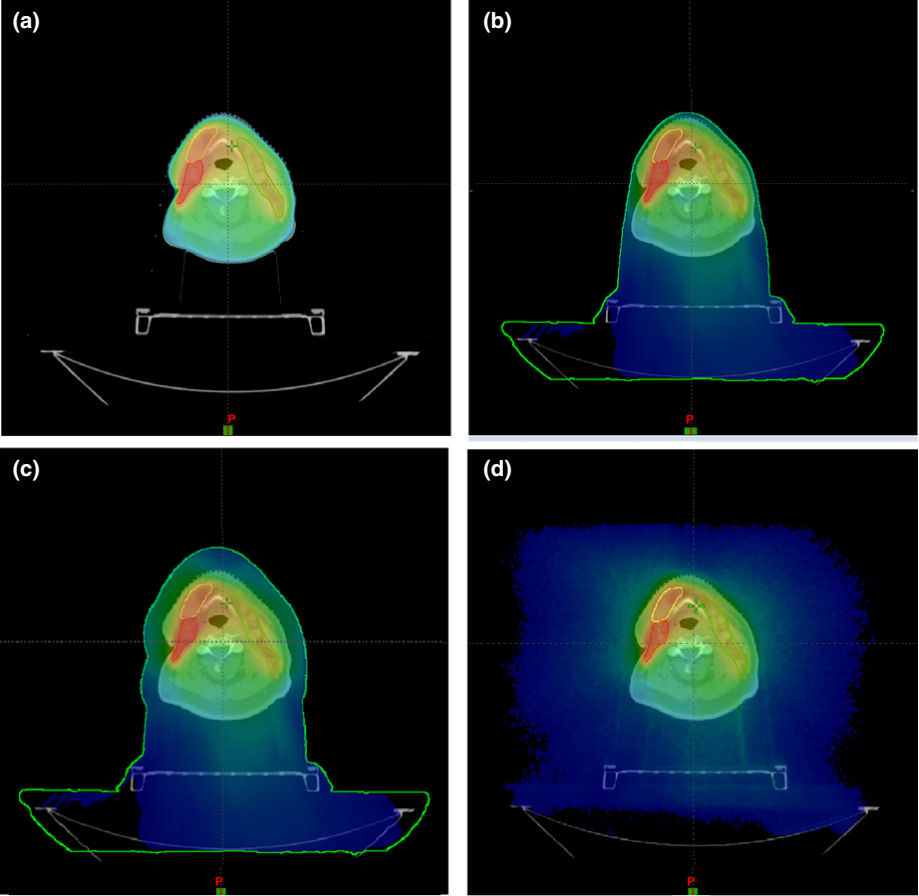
An axial view of patient comparison of dose distributions for an H&N cancer patient treated with 6 MV VMAT beams. (a) Eclipse with default body contour; (b) Eclipse with external body contour including head mask and table; (c) Eclipse with an extended external body contour including table; (d) Monte Carlo calculated with CT images including table.
